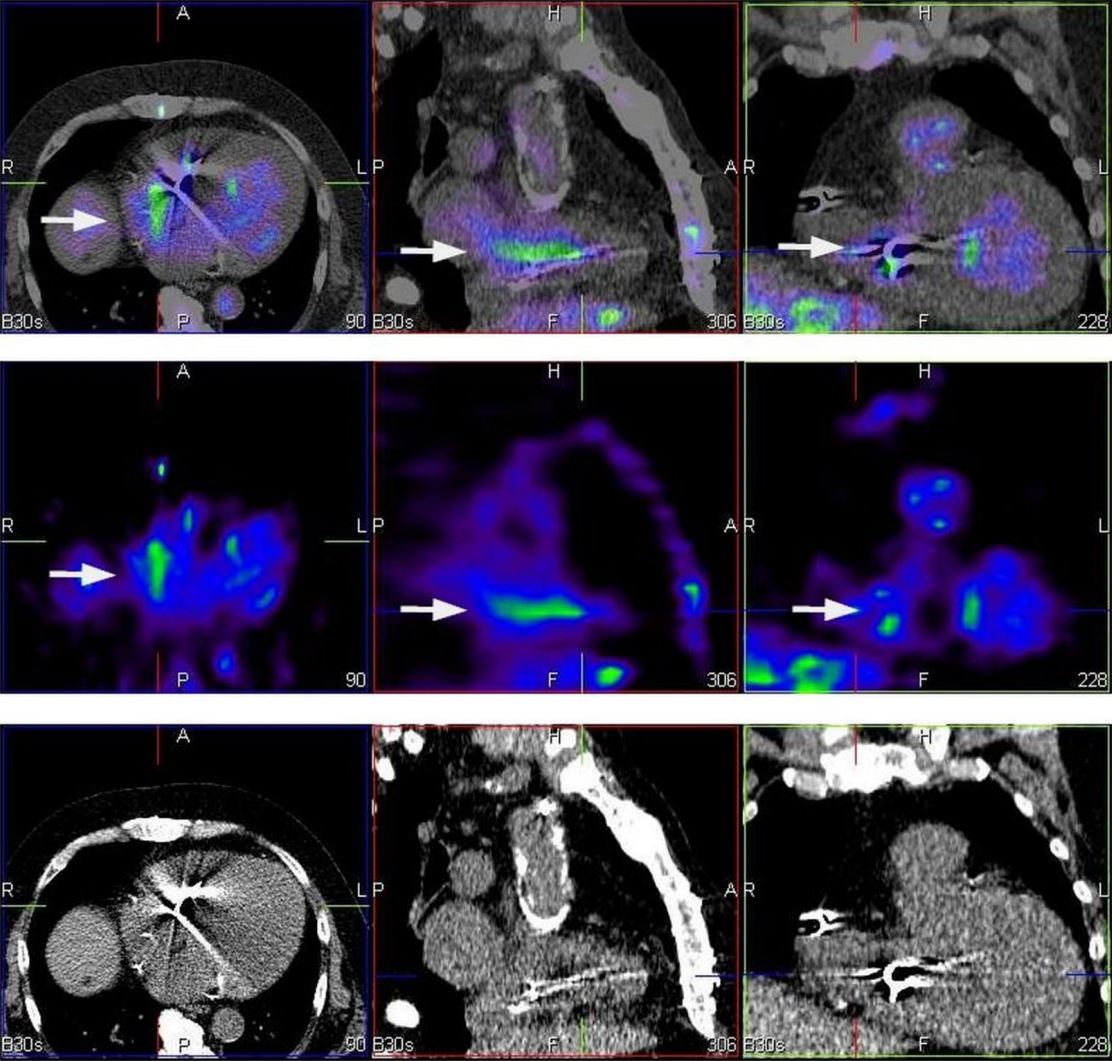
99mTc-HMPAO-SPECT/CT—study in three projections axial (left), sagittal (middle) and coronal (right). Bottom panel presents CT scans. Corresponding SPECT sections are presented in middle row and fusion images (99mTc-HMPAO-SPECT/CT) are placed in upper row. 99mTc-HMPAO-SPECT/CT shows an accumulation of radiolabeled leucocytes in the vicinity of an implanted electrode (arrows). In this case tracer uptake is consistent with CDRIE
